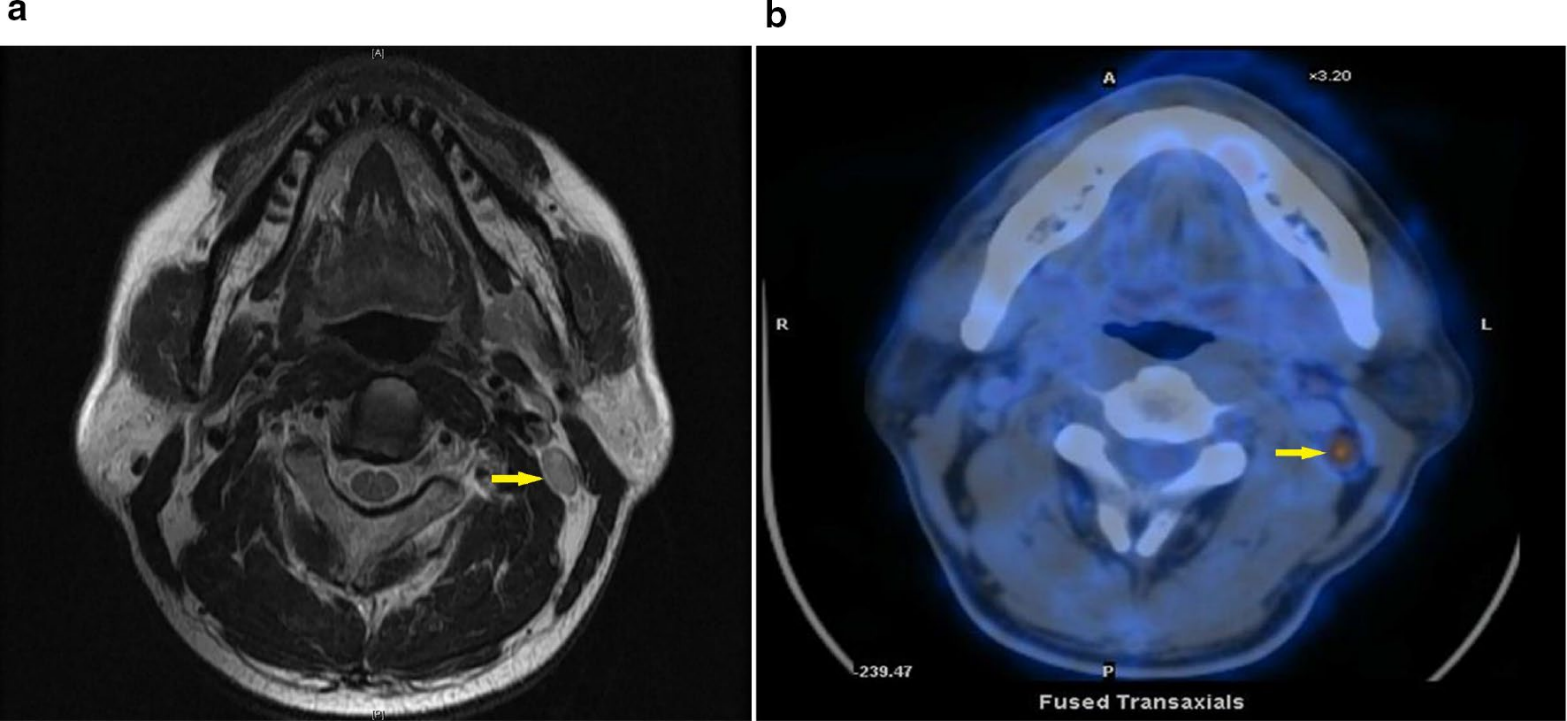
A 35-year-old man with a SCLN of 7.5 mm (arrow) in left level IIb was diagnosed with T3N0 nasopharyngeal cancer by MRI (a), but diagnosed with T3N1 disease by PET/CT (b). *SCLN* small cervical lymph node, *MRI* magnetic resonance imaging, *PET/CT* positron emission tomography/computed tomography
